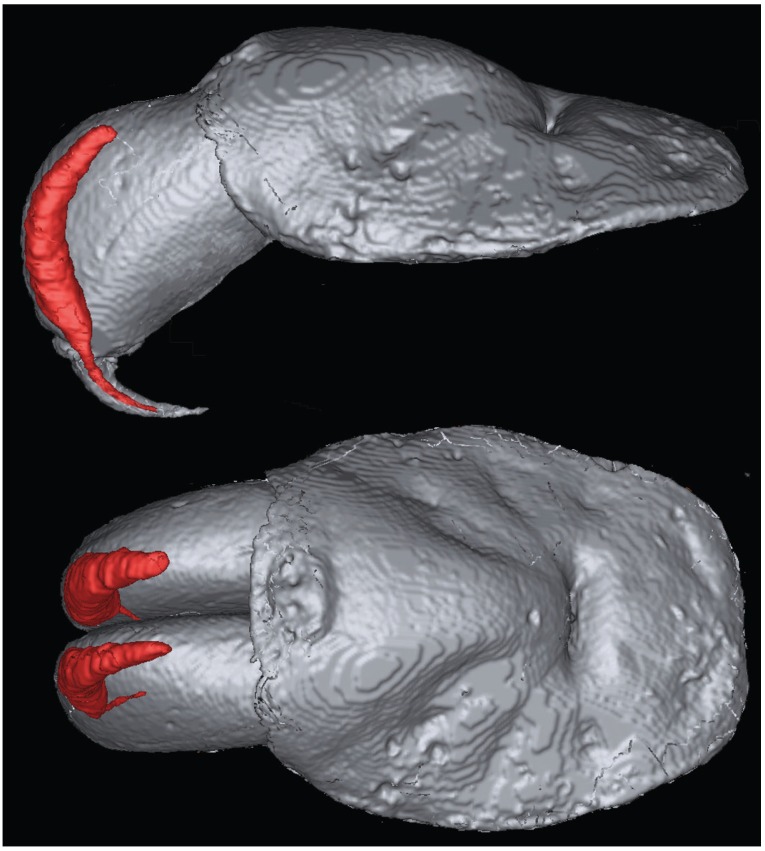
Magnetic resonance imaging of *Trittame loki* venom glands.
